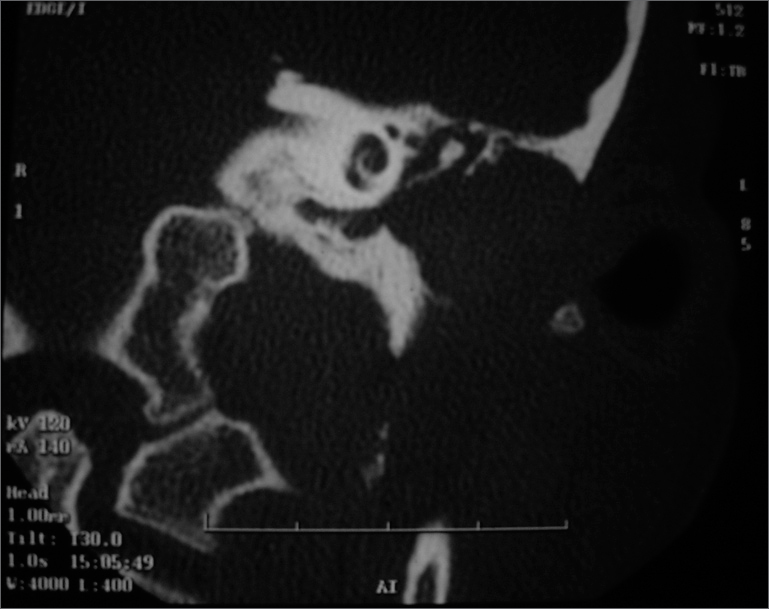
Temporal bone CT Scan - Left Ear.
